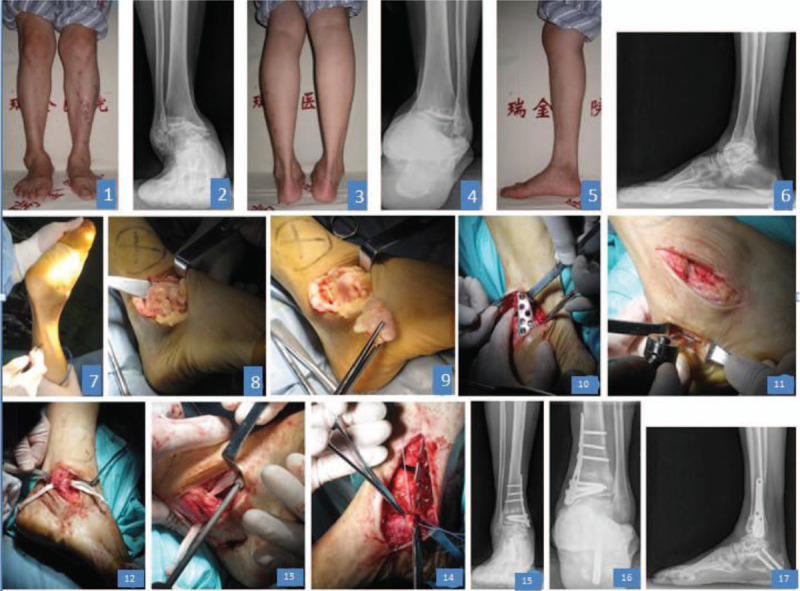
A 69-year female patient with right ankle injury for 18 years and aggravation for 5 years; (A–F) normal and lateral X-rays of right ankle and metaleg force line, grade 3b varus ankle arthritis, and metaleg varus deformity; (G) Achilles tendon was prolonged; (H–J) external osteophyte was cleaned, and the anterior talofibular ligament was loosened and dysfunctional; (J) open osteotomy of the internal distal tibia which was fixed by the steel plate; (K) calcaneus diagonal osteotomy was carried out and emigration, and 2 cannulated screws (6-7 mm in diameter) were fixed; (I) allogenic ligament was collected for reconstruction through the distal tibia bone tunnel; (M) lateral ligament and talus were fixed on the neck, and lateral calcaneus on body with compression screws (7 × 23 mm); (N) the internal lateral ligament was prolonged and sutured; (O–Q) normal and lateral X-rays indicating the correction of the varus ankle and metapodium.
